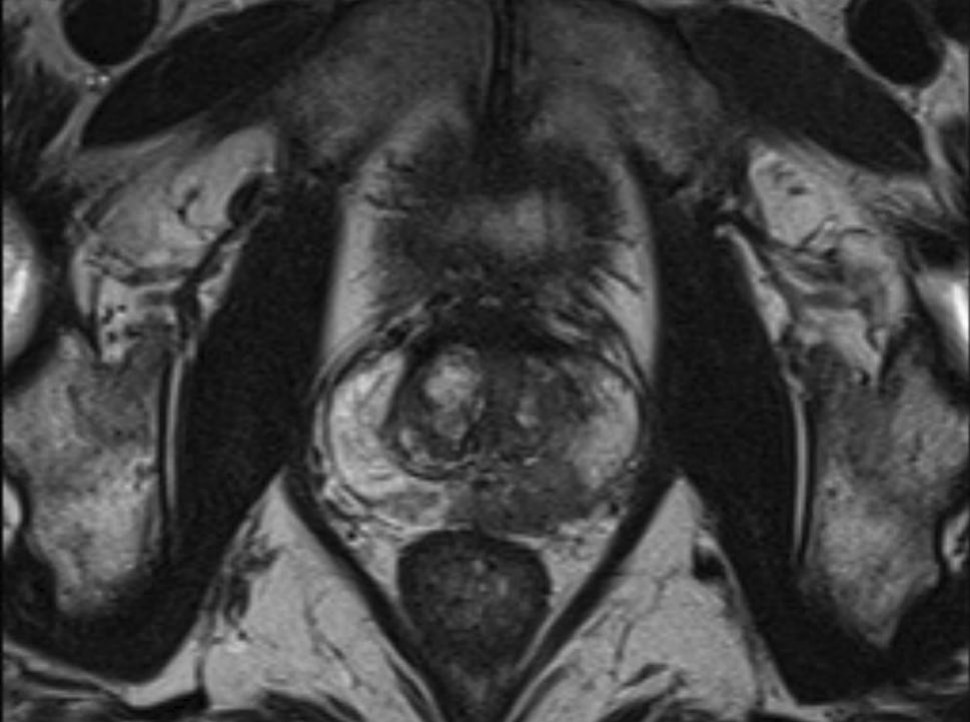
An axial T2-weighted image on a 3 T prostate MRI demonstrating a PI-RADS 5 lesion in the left peripheral mid gland
